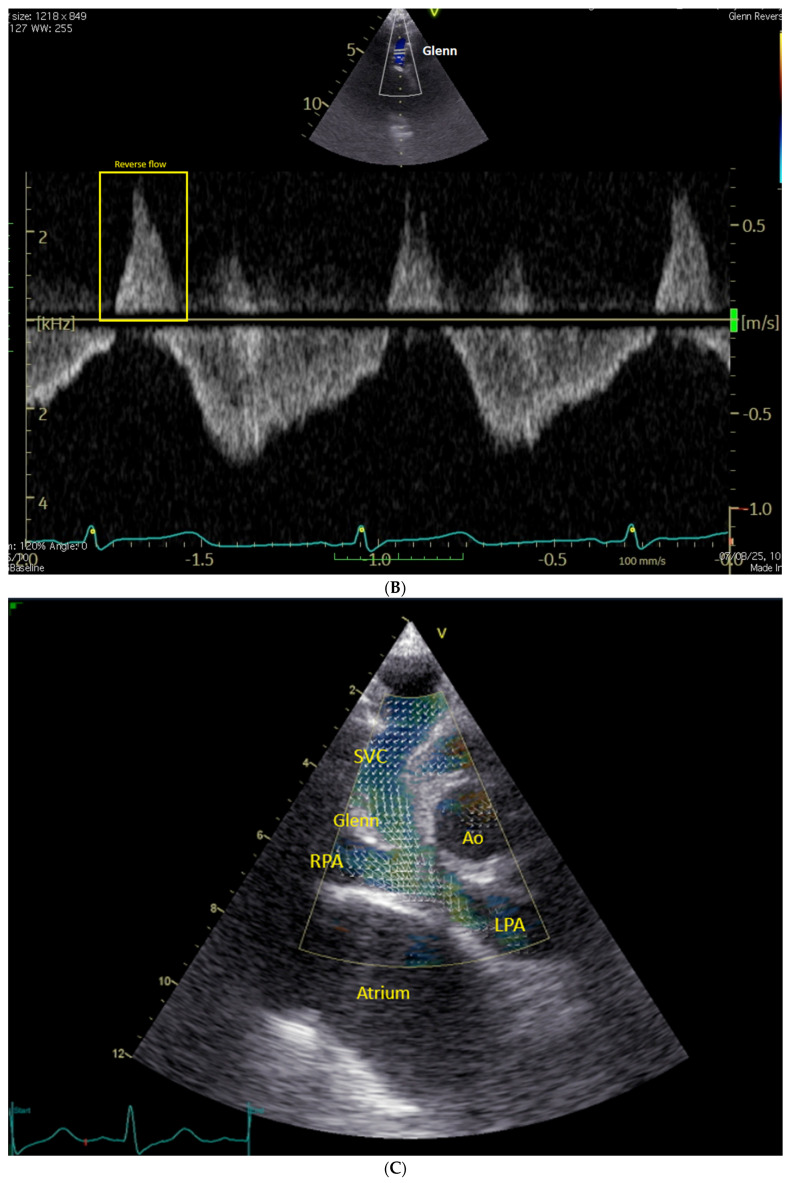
(A): normal, phasic, and low-velocity Doppler flow in Glenn; (B): reverse flow in Glenn; (C): blood flow in Glenn and pulmonary arteries are visualized by BST vector flow reconstruction. Ao = aorta, LPA = left pulmonary artery, RPA = right pulmonary artery, SVC = superior vena cava.
